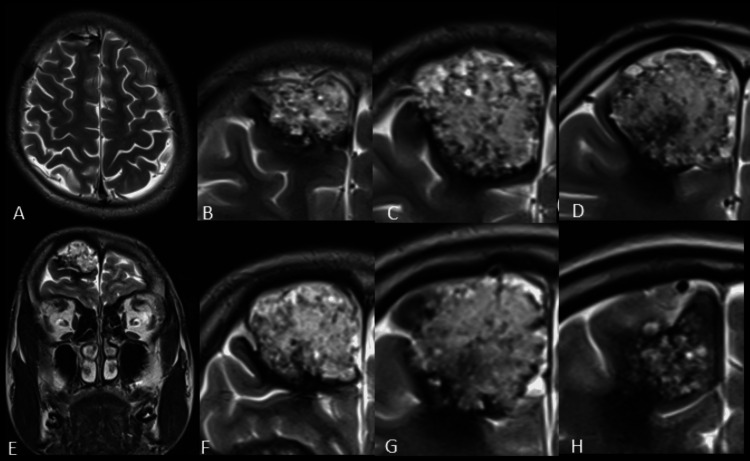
T2 weight axial (A-D) and coronal (E-H) MRI images. Images showed a well-defined heterogeneously hyperintense cortex-based lesion in the anterior frontal lobe in the right parasagittal plane.
